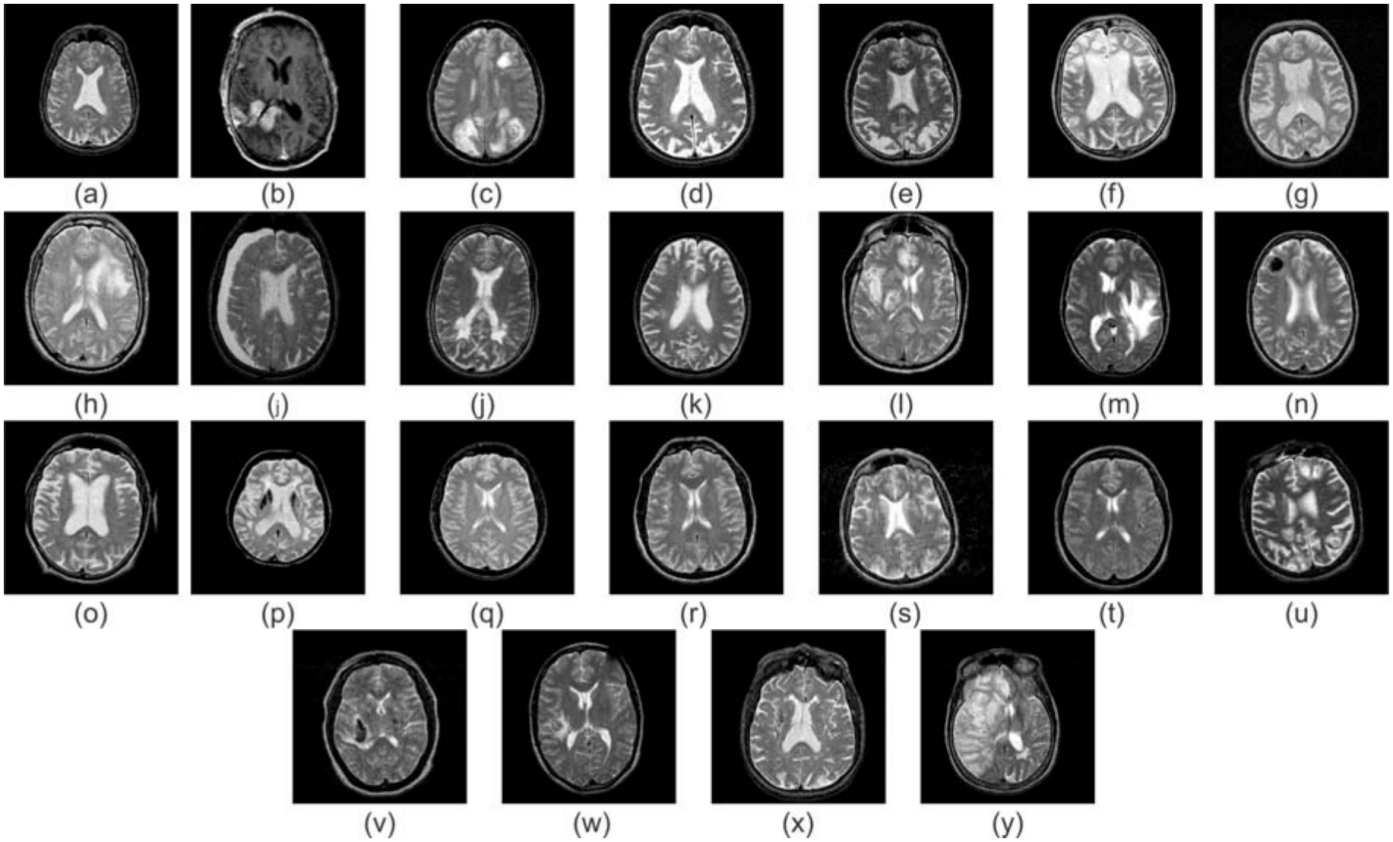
Sample images of various diseases in brain MRI dataset: (a) Normal brain (b) Glioma (c) Sarcoma (d) Alzheimer’s disease (e) Alzheimer’s disease with visual agnosia (f) Pick’s disease (g) Huntington’s disease (h) Meningioma (i) Chronic subdurnal hematoma (j) Multiple sclerosis (k) Cerebral toxoplasmosis (l) Herpes encephalitis (m) Metastatic bronchogenic carcinoma (n) Metastatic adenocarcinoma (o) Motor neuron disease (p) Cerebral calcinosis (q) AIDS dementia (r) Lyme encephalopathy (s) Creutzfeld-Jakob disease (t) Hypertensive encephalopathy (u) Multiple embolic infarctions (v) Cerebral haemorrhage (w) Cavernous angioma (x) Vascular dementia (y) fatal stroke.
